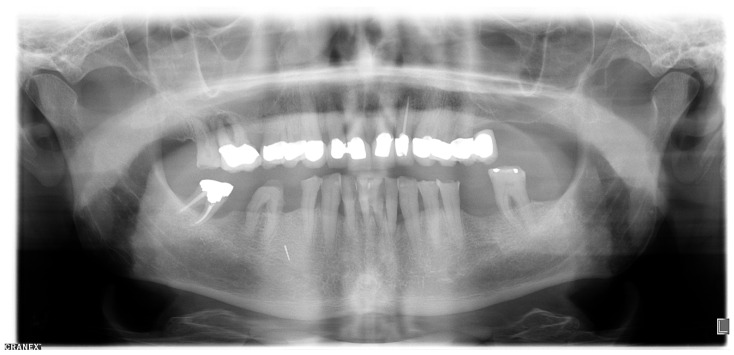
Orthopantomogram taken in October 2019.
